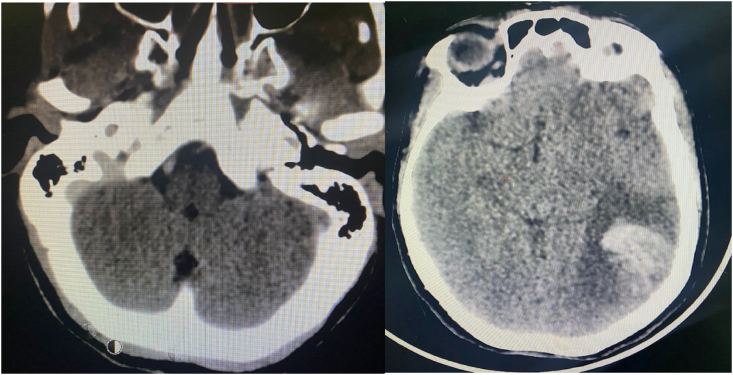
CT image showing left hemorrhagic infarction over left lateral sinus thrombophlebitis.
